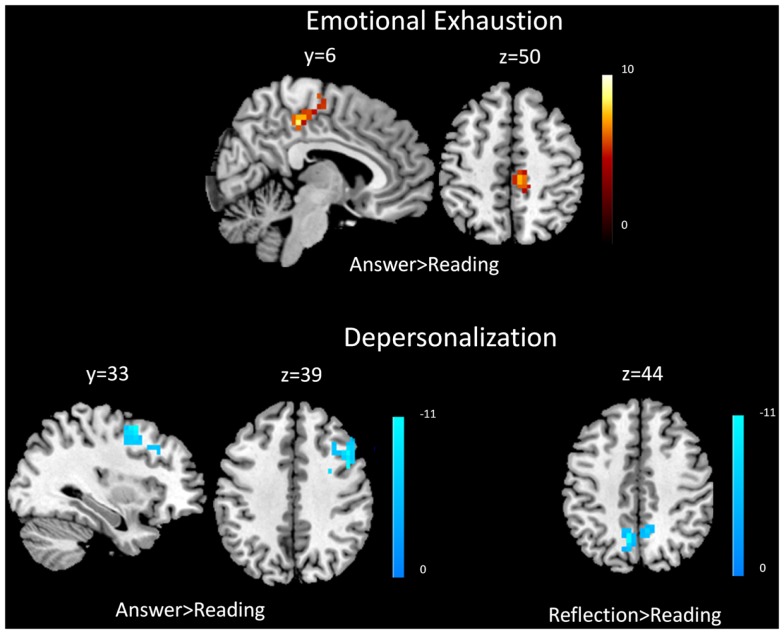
Functional magnetic resonance imaging results. Covariate results for emotional exhaustion and the beta values of the answering > reading contrast (top panel) for residents. Covariate results for depersonalization and answering > reading contrast (left) and reflection > reading contrast (right) for residents (bottom panel). The color bar denotes the *t*-scores of the estimated slope of the beta values vs. the covariate.
